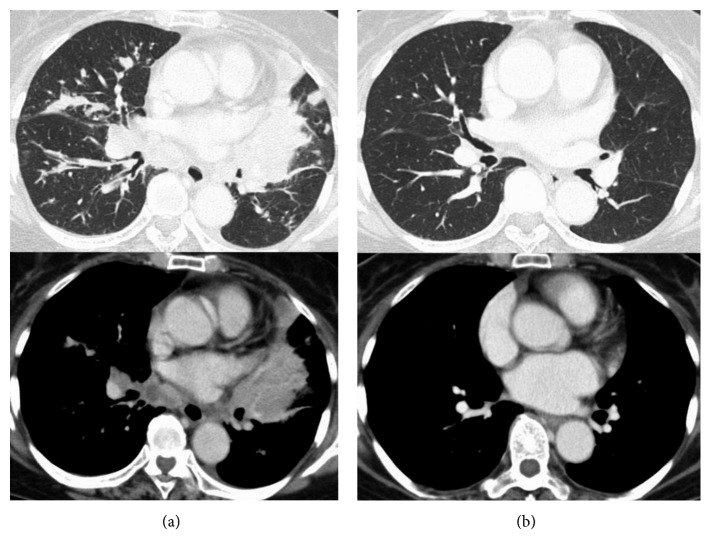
CT findings before and after the treatment. (a) Chest CT at admission. It showed a tumor in the left lower lobe of the lung with mediastinal lymphadenopathy, pleural dissemination, and multiple pulmonary metastases in both of the lungs. (b) CT revealed dramatic tumor reduction in the primary and metastatic lesions, and this had maintained after 9 months since we started the treatment.
